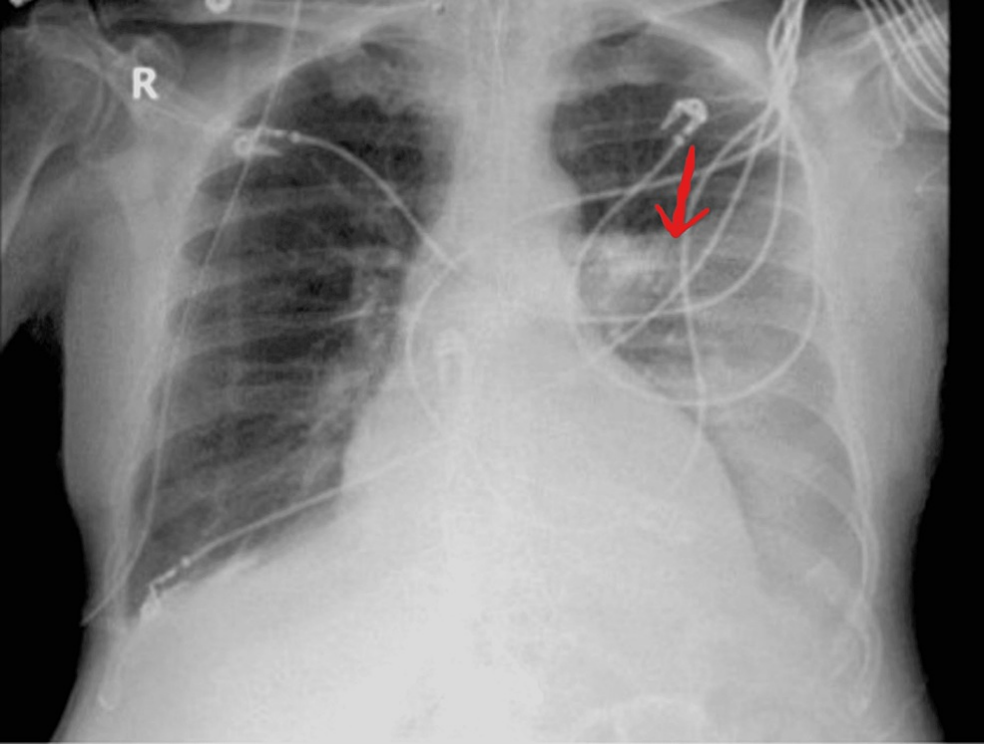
Chest x-ray showing left-sided pleural effusion (red arrow)
